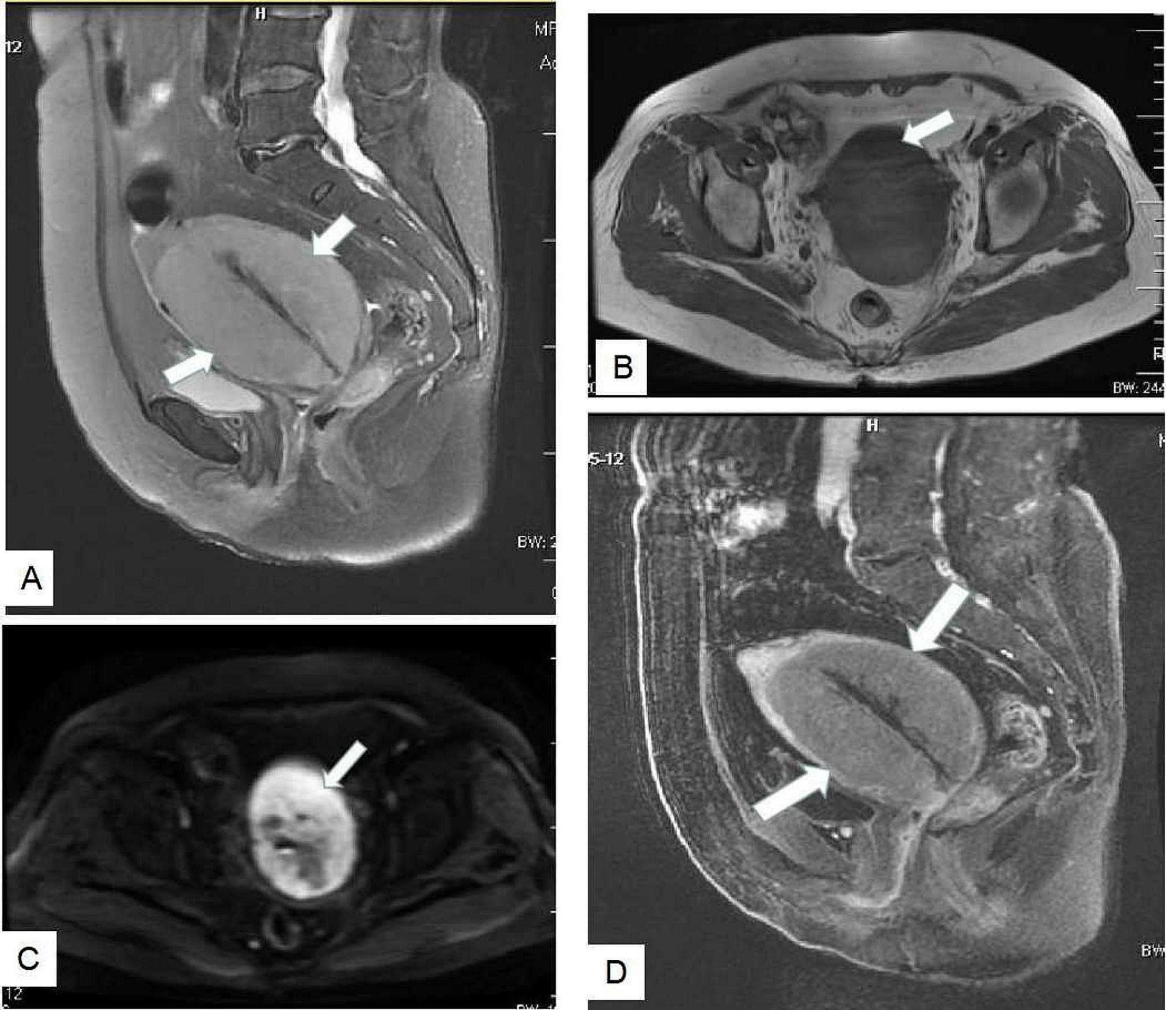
MRI images: (A) Sagittal T2-weighted image: enlarged homogeneous mass of uterus shows intermediate intensity. (B) Axial T1-weighted image: a large homogenous uterus mass exhibits intermediate signal intensity similar to the muscle. (C) Axial DWI image: the uterus mass presents restricted diffusion with high signal intensity. (D) Postgadolinium sagittal T1-weighted image: mild homogenous enhancement of the uterus mass after gadolinium administration (Case 9)
